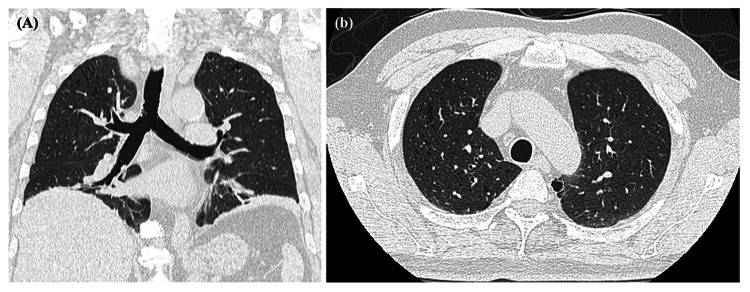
Chest CT of the patient. The chest CT showed no abnormalities in the pleural field. The CT did not include the periscapular muscles within the scanning window.
